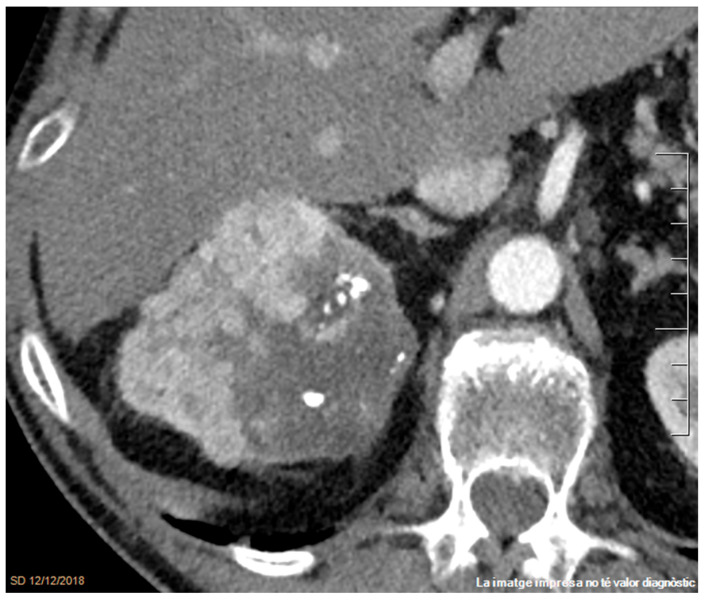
Infiltrating right clear cell RCC with calcifications. A contrast-enhanced axial CT image in the venous phase demonstrates a hypervascular mass with calcifications and heterogeneous enhancement due to the presence of a large non-enhancing necrotic area. Peritumoral vessels are visible within the perirenal space.
